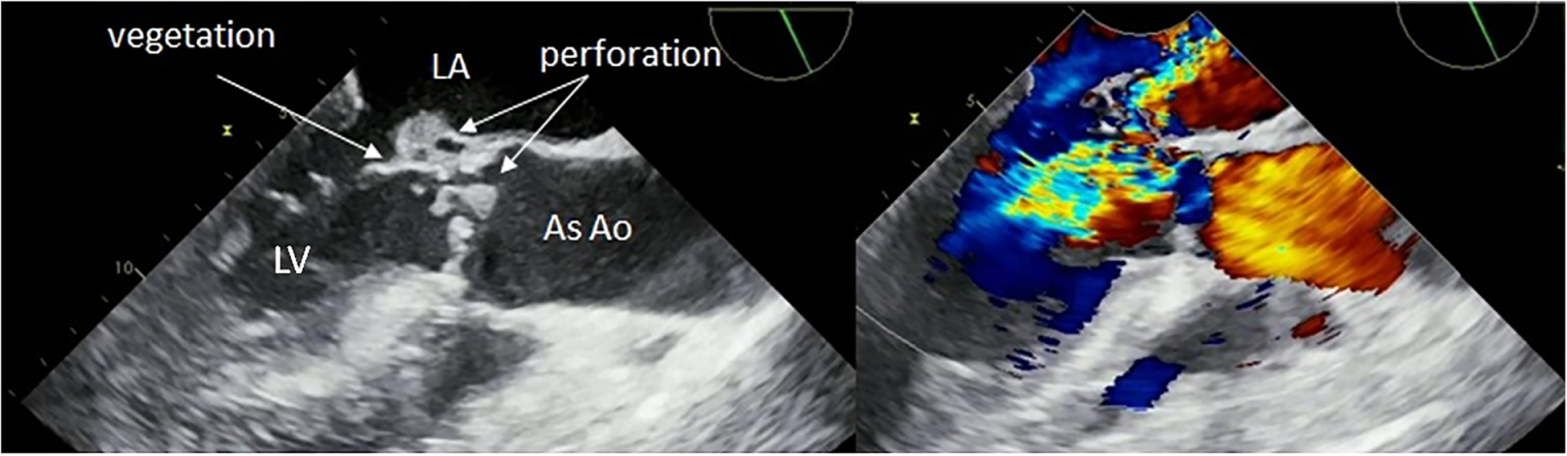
Three-chamber transoesophageal echocardiographic view reveals vegetation (size, 25 × 15 mm) attached to the anterior leaflet of the mitral valve. Color flow imaging reveals severe insufficiency of both the aortic and mitral valves with perforation in the AMC and at the base of the non-coronary cusp. LV left ventricle, LA left atrium, AsAo ascending aorta, AMC aorto-mitral curtain
